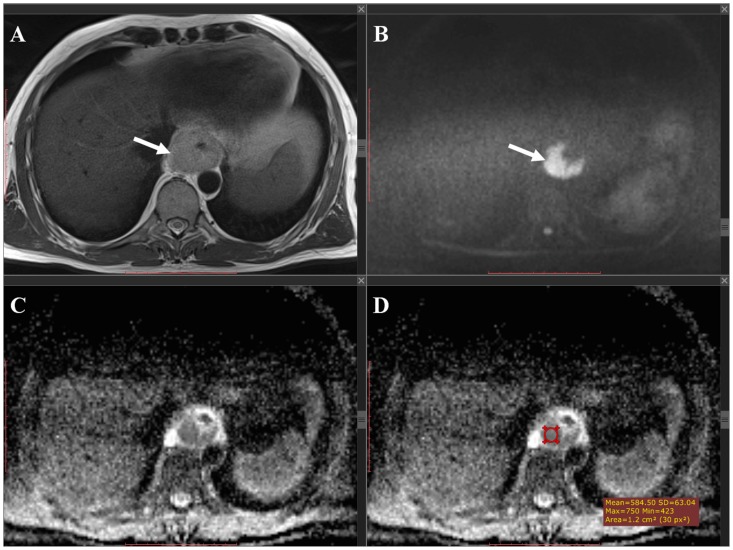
Manual measurement method. T2-weighted (A) and b1000 DW (B) images, and corresponding ADC map (C and D). An esophageal tumor can be depicted as thickening of the esophageal wall (arrow in (A) with high signal intensity on the corresponding b1000 DW image (arrow in B) and low signal intensity on the corresponding ADC map (arrow in C). An ROI was manually drawn in the tumor on the ADC map (red circular region in D) to calculate the tumor ADC value.
